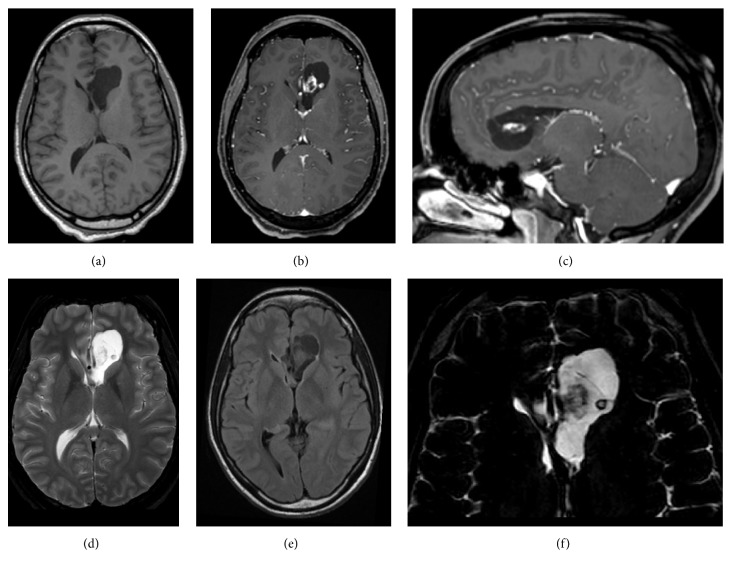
Precontrast axial (a), postcontrast axial (b), and postcontrast left parasagittal (c) T1-weighted MR images of the brain demonstrate an intra-axial left frontal mass with heterogeneous internal nodular and septal enhancement. The largest enhancing component is subjacent to markedly thinned medial left frontal cortex. T2-weighted axial images (d) demonstrate hyperintense cystic formation with near complete suppression on FLAIR sequences (e). No peritumoral T2 hyperintensity is present to suggest vasogenic edema or gliosis. Details from high-resolution, heavily T2-weighted images (f) redemonstrate mixed solid and cystic components and thin internal septation. No elevated perfusion or abnormally restricted diffusion was identified on MR perfusion or diffusion-weighted imaging, respectively (not shown).
